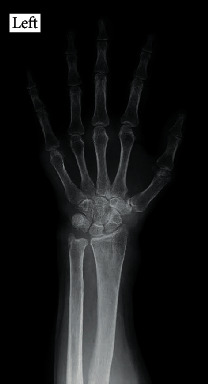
Anterior-posterior plain radiographs showing bone atrophy around the wrist and the metacarpophalangeal joints.
